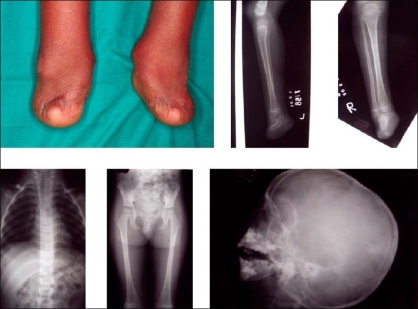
Feet deformities with X-ray of lower limbs, pelvis, clavicles, and skull
